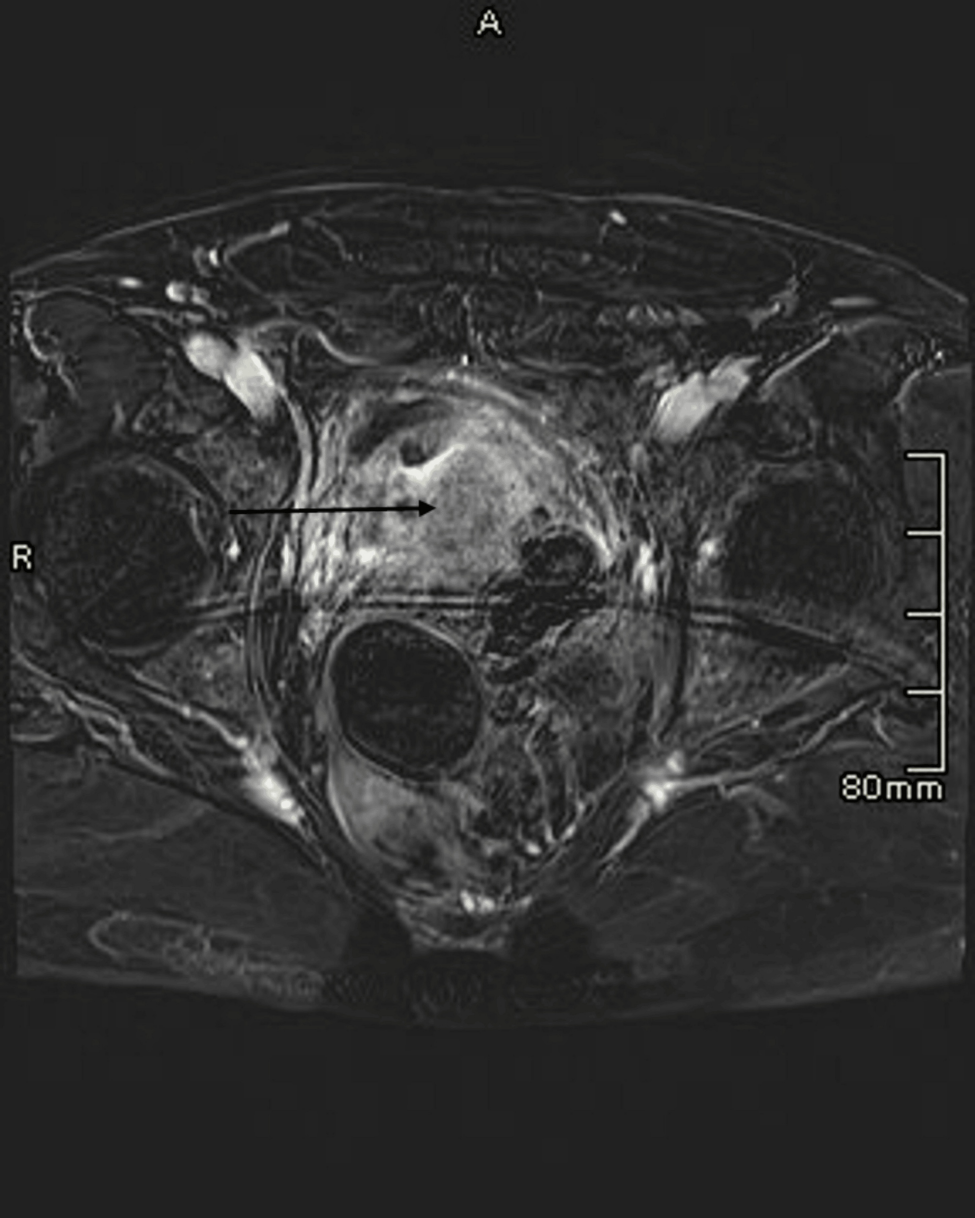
Prostate MRI Multiple non-enhancing T1 hyperintense, heterogeneous T2 signal intensity cystic lesions in the pelvis. The lesions appear to arise from the posterior wall of the prostate and exert a mass effect on the left anterolateral wall of the rectum. No definite communication with the rectal lumen. MRI: magnetic resonance imaging
